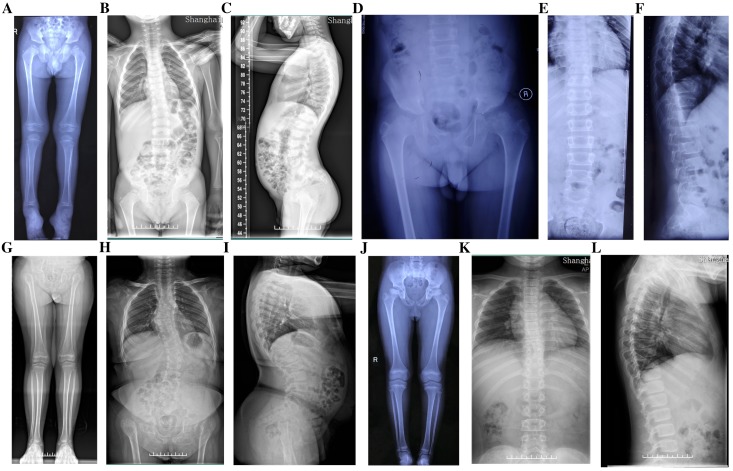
Radiographs of the probands with SEDC. A-C: Full-length X-rays of both lower extremities (A) and spine radiographs (B, C) of the proband in Family 1. Flattening of the acetabular roof, small capital femoral epiphyses, enlarged distal femur metaphyses and ovoid vertebral bodies were displayed. D-F: X-rays of pelvis (D) and spine (E, F) of the proband in Family 2. Partial collapse and uneven density of bilateral femoral heads, flattening of the acetabular roof, shortening of the femoral neck and platyspondyly were shown. G-I: Full-length X-rays of both lower extremities (G) and spine radiographs (H, I) of the proband in Family 3. Small iliac bones, horizontal acetabular roof, coxa vara, very short femoral necks, small and fragmented ossification of capital femoral epiphyses, enlarged distal femur metaphyses, severe scoliosis and moderate platyspondyly were demonstrated. J-L: Full-length X-rays of both lower extremities (J) and spine radiographs (K, L) of the proband in Family 4. Flattening of the acetabular roof, compressed femoral head, shortening of the femoral neck and base of the ilium, distinctly flared metaphyses of femurs and slight platyspondyly were shown.
